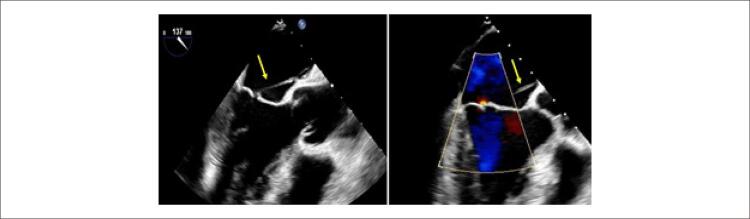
Imagens 2D de ecocardiograma transesofágico que mostram estrutura fina e bem delineada (seta amarela), com origem no septo interatrial até à ponta do folheto anterior da valva mitral. Ausência de regurgitação significativa através de Doppler cor (à direita).
